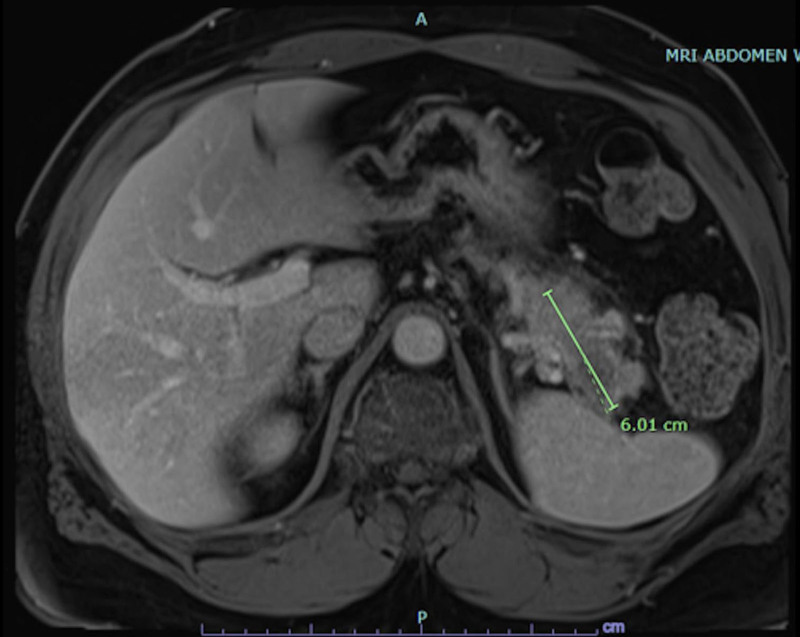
Magnetic resonance imaging of the abdomen with and without contrast showing segmental enlargement of the pancreatic tail.
